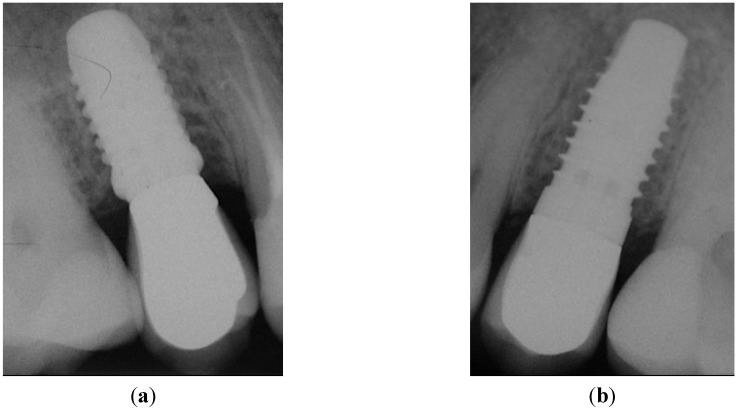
Peri-apical X-rays taken after AC. (a) Position 15; (b) Position 25. No pathological signs were visible around both implants.
